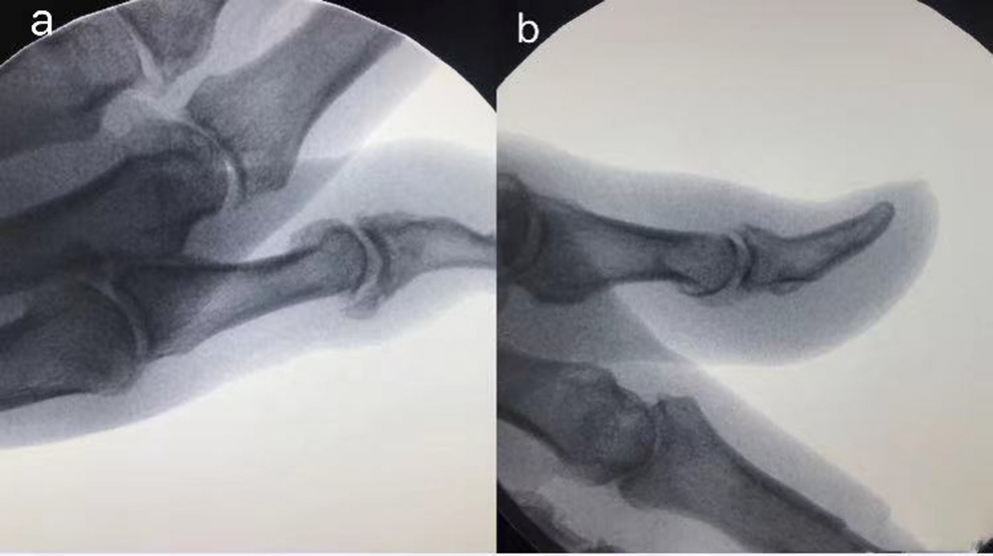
The imaging examination of the patient before and after surgery. (a) Pre-operative X-ray showed a large number of osteophytes in the DIP joint of the thumb; (b) X-ray showed osteophyte disappeared 3 months after the operation.
